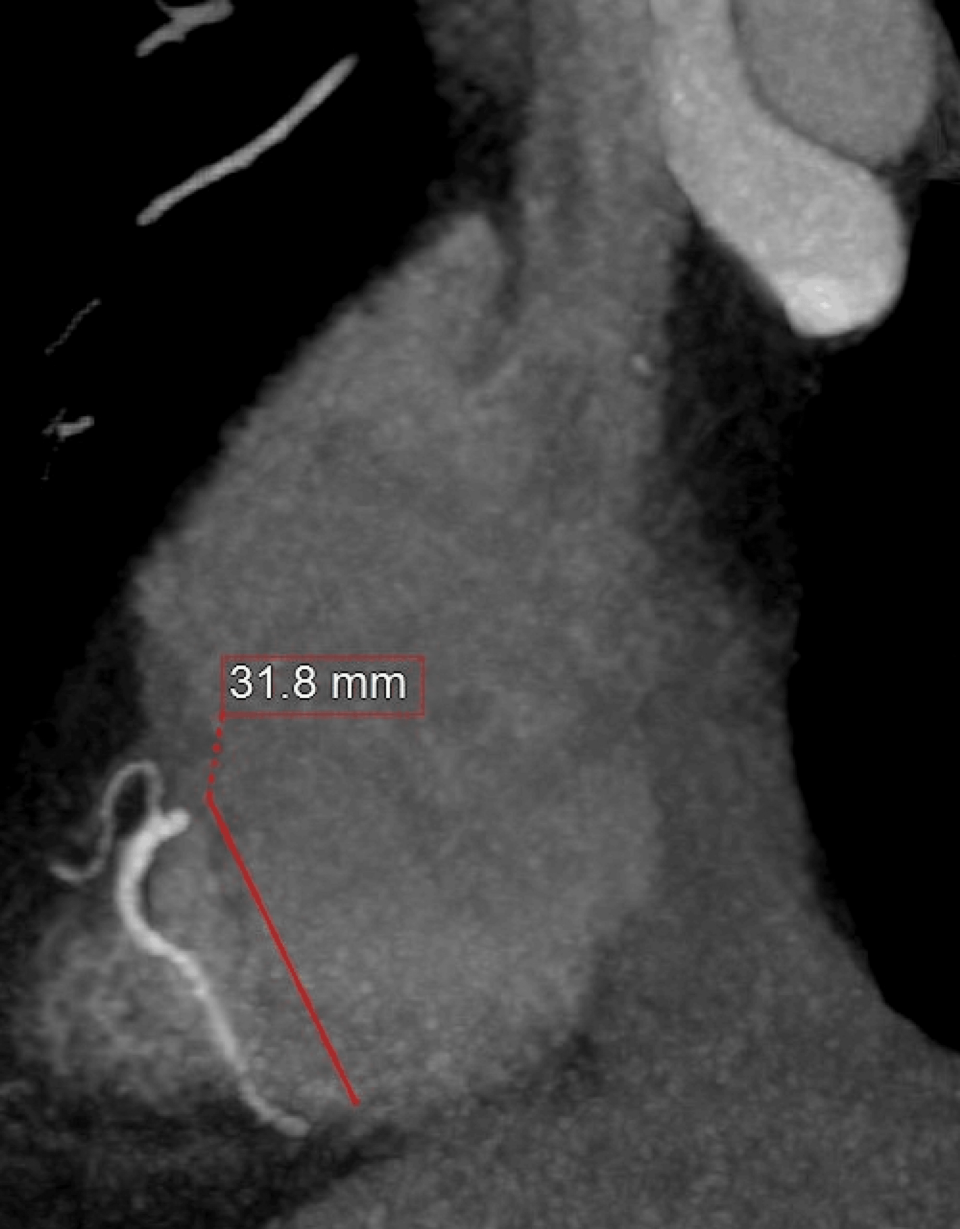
Multi-detector computed tomography angiography (MDCTA) showing the intra-atrial segment of the RCA (31.8 mm) The artery traverses the right atrium before resuming its epicardial course. MDCTA: multi-detector computed tomography angiography, RCA: right coronary artery
